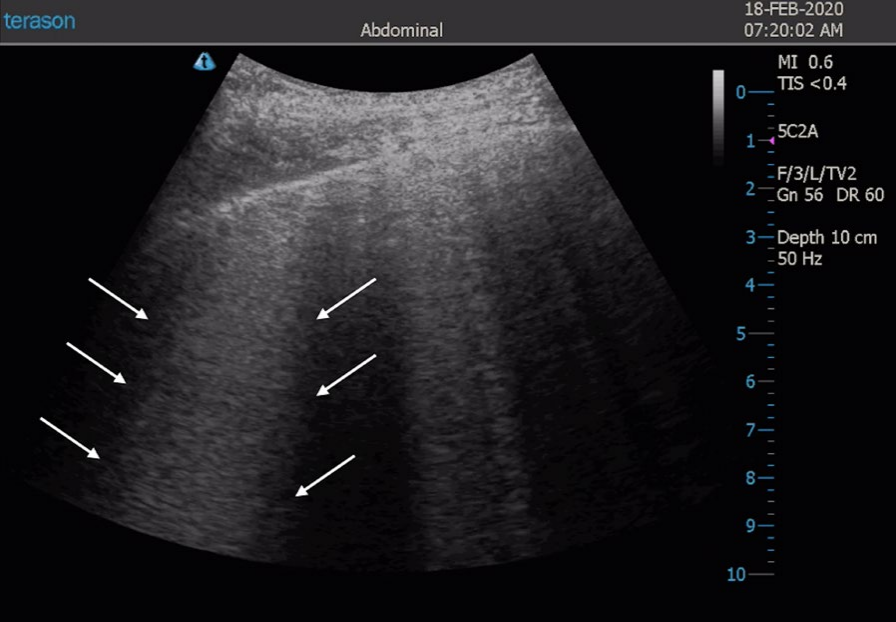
Sonographic appearance of coalescent B-lines. White arrows indicate area with coalescent B-lines, called white lung
